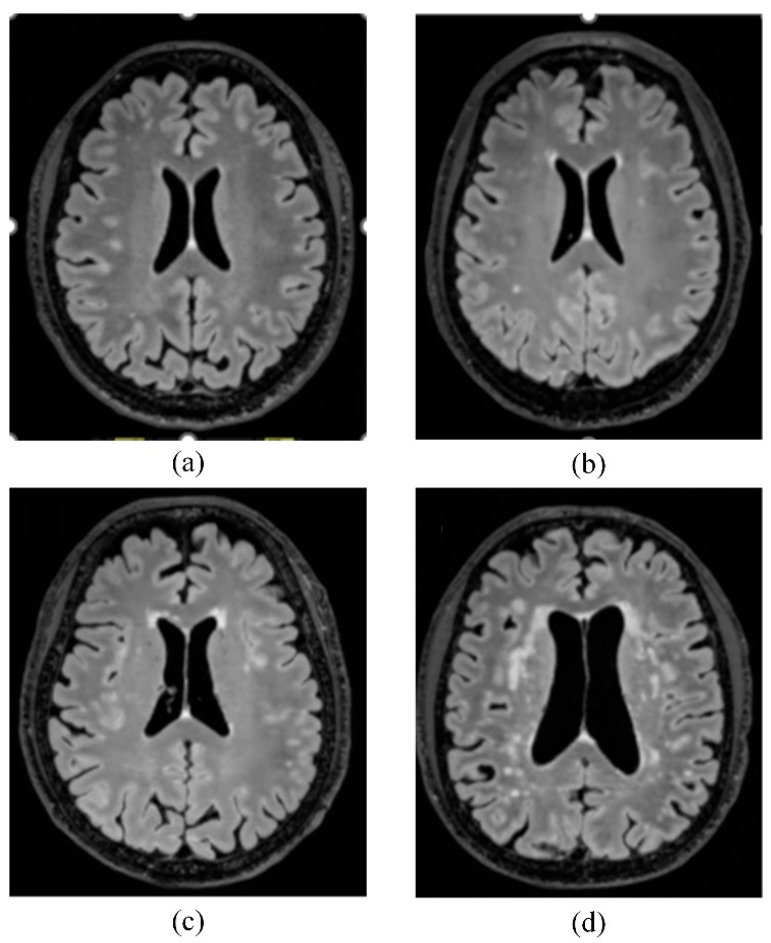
Representative cases of the different degrees of white matter lesions classified by the Fazekas scale on FLAIR. (a) Absent WMH, no visible white matter hyperintensities; (b) mild WMH, “caps” or pencil-thin lining hyperintensities visualized on periventricular white matter region and punctate foci on deep white matter region; (c) moderate WMH, beginning confluence on deep white matter region or smooth “halo” hyperintensities on periventricular white matter region; (d) severe WMH, irregular periventricular hyperintensities extending into the deep white matter.
